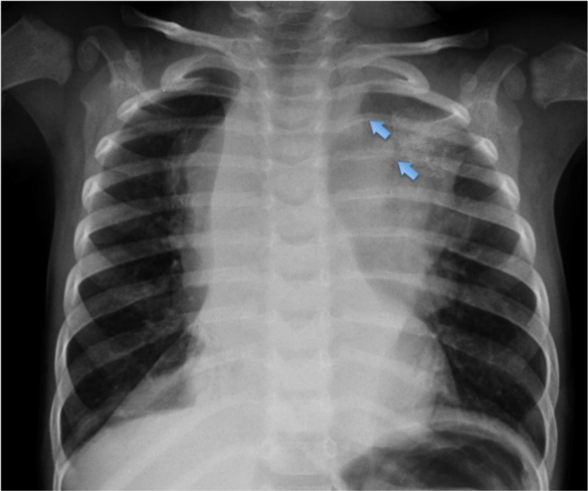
Chest x-ray of 3 year old girl showing thoracic NBL. Note erosions of the posterior 3
